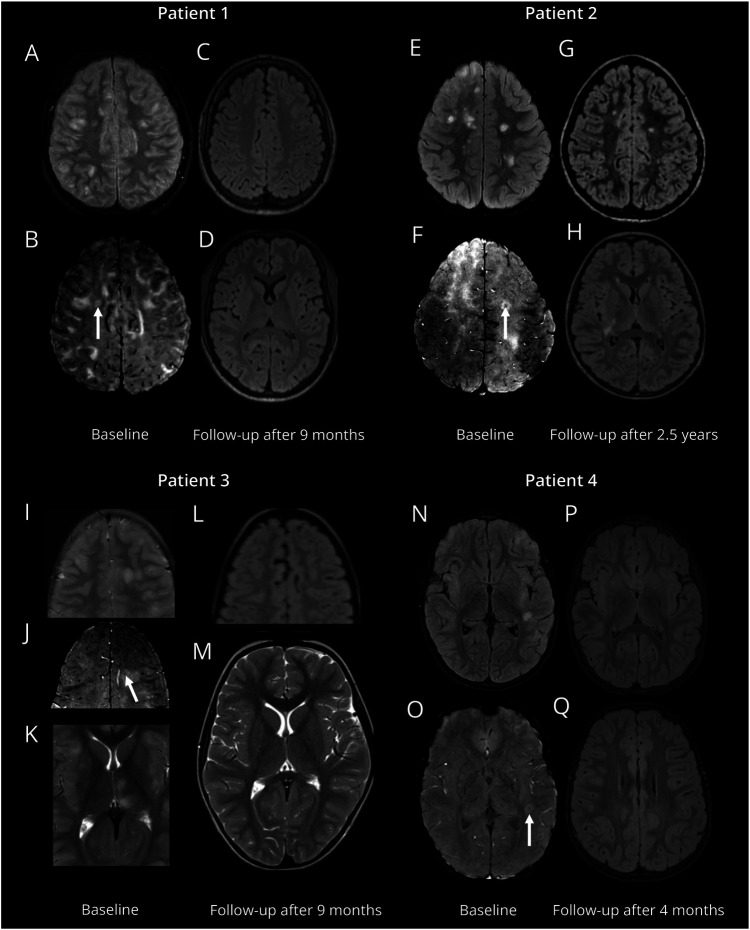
Imaging Findings in Patients With ≥6 CVS+ Lesions MRI scans from 4 patients (P1, P2, P3, P4) demonstrating reference/initial scan and follow-up evaluations. P1: Reference/initial scans include T2-weighted imaging (A) and SWI (B), with a white arrow highlighting a CVS+ lesion. Follow-up scans 9 months after (C, D) show complete resolution of the lesion. P2: Reference/initial scans include T2-weighted imaging (E) and SWI (F), with a white arrow pointing to a CVS+ lesion. Note the presence of thalamic lesions (K). Follow-up scans 2.5 years after (G, H) show persistence of CVS+ lesion. P3: Reference/initial scans include T2-weighted imaging (I, K) and SWI (J), follow-up scans 9 months after (L, M) demonstrate complete resolution of all lesions. P4: Reference/initial scans include T2-weighted imaging (N) and SWI (O), with a white arrow marking a CVS+ lesion. Follow-up scans 4 months after (P, Q) show resolution of the lesion. CVS = central vein sign; SWI = susceptibility-weighted imaging.
